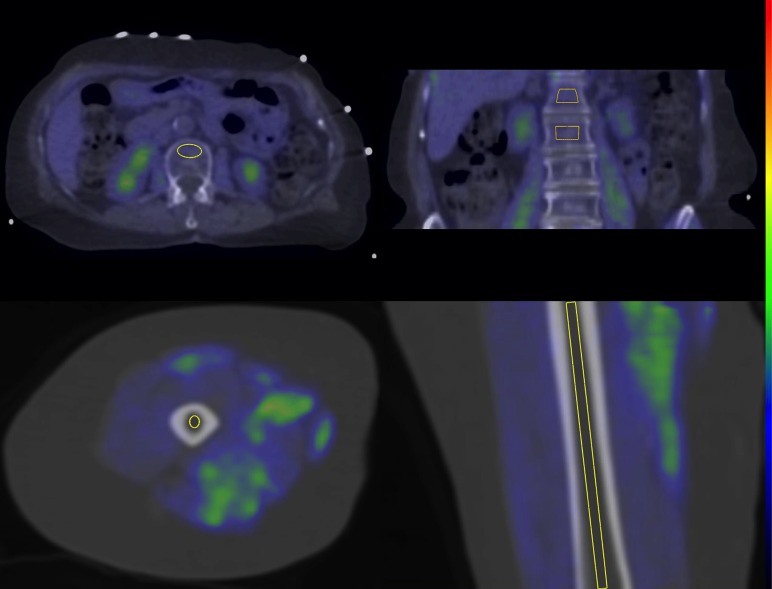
Cylinder-shaped volumes of interests of vertebral (upper row) and femoral bone marrow (lower row) in transaxial and coronal PET/CT-images.
